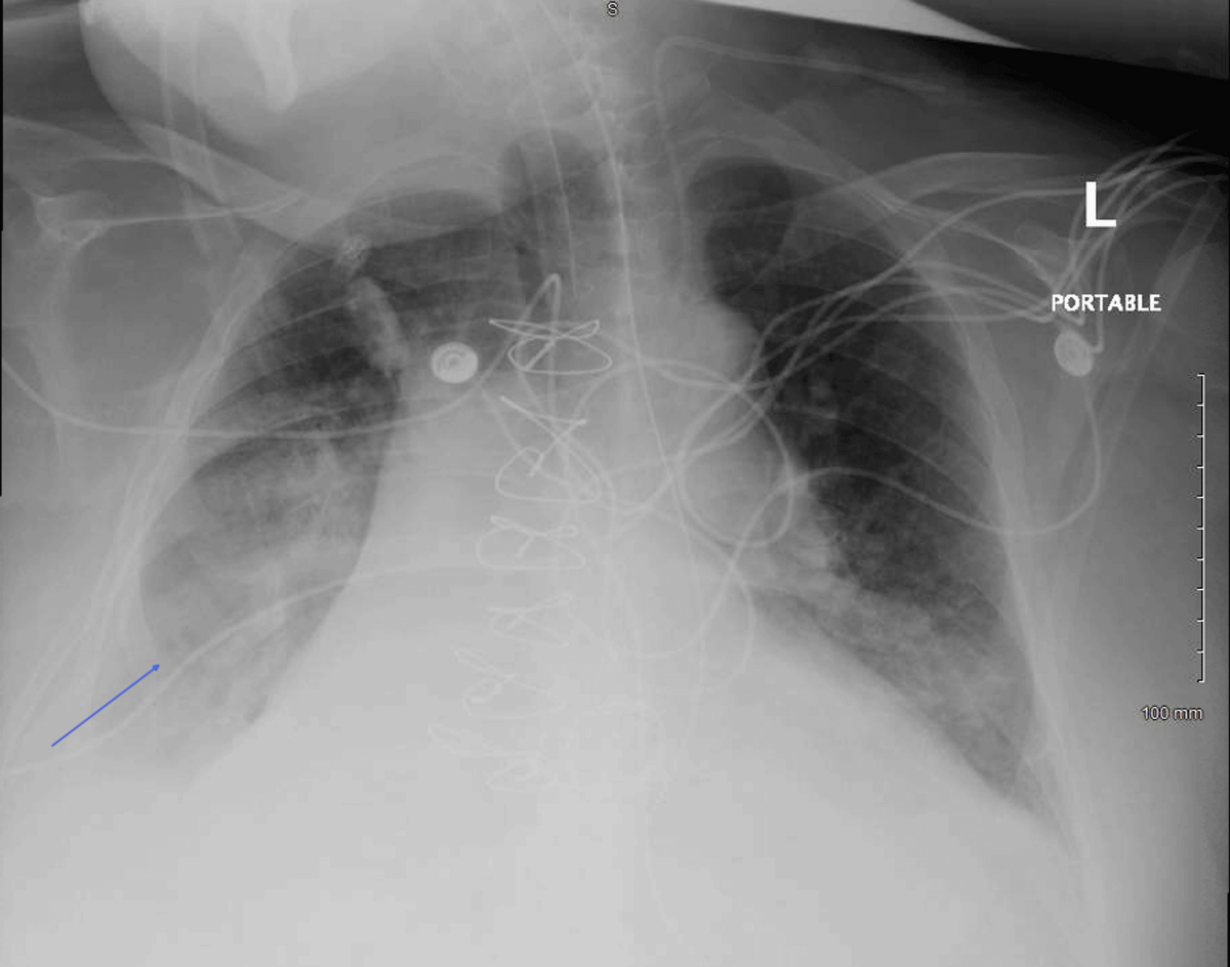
Portable chest X-ray showing right basilar infiltrate
